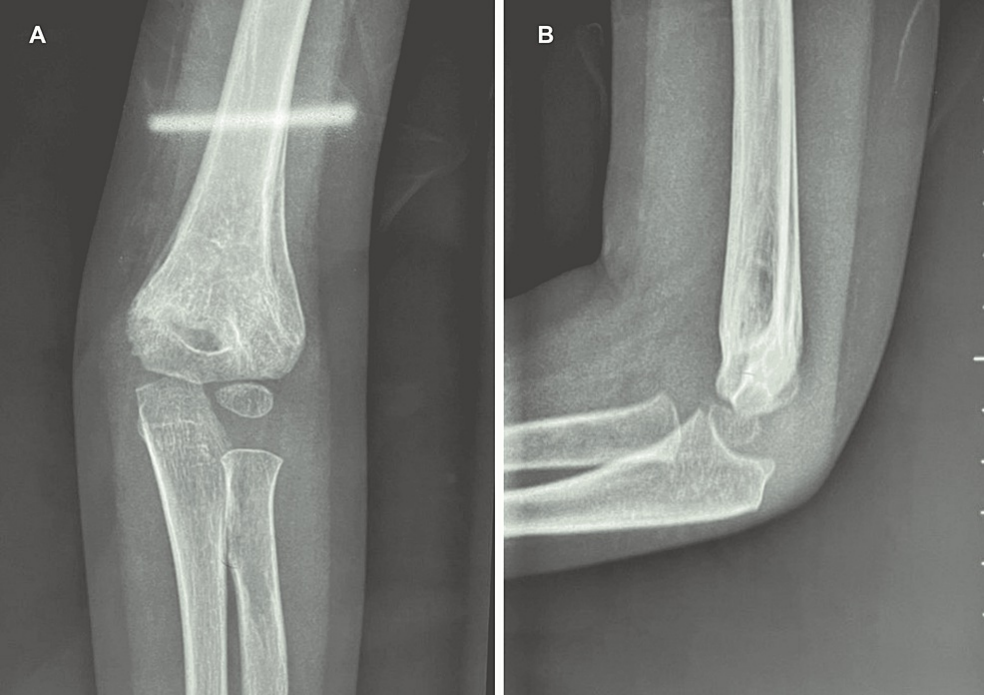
Postoperative x-ray in the anteroposterior view (A) and lateral view (B) of the elbow.
